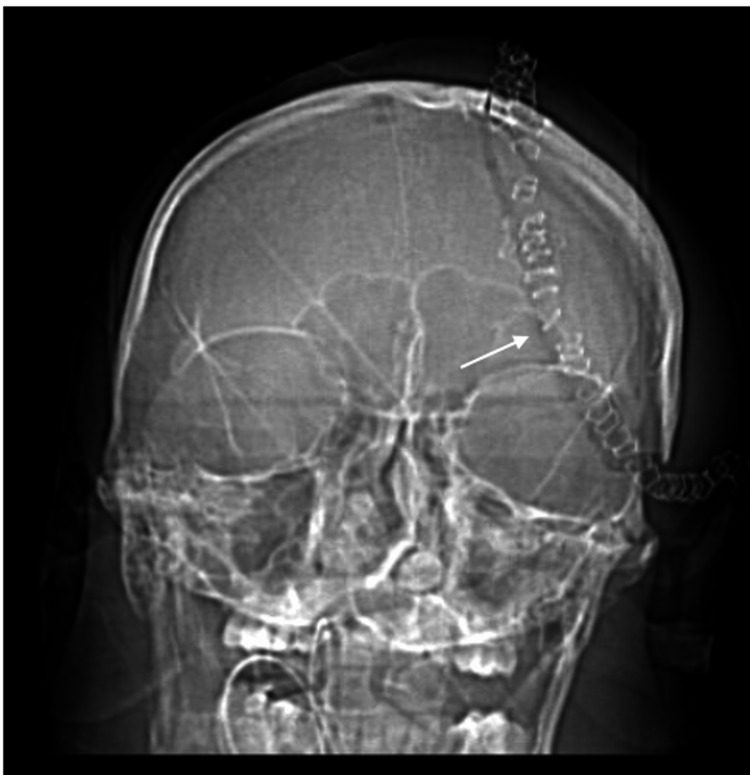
AP CT scout image showing that the craniotomy flap has hinged outward. Image courtesy Dr. Salazar Jones.
